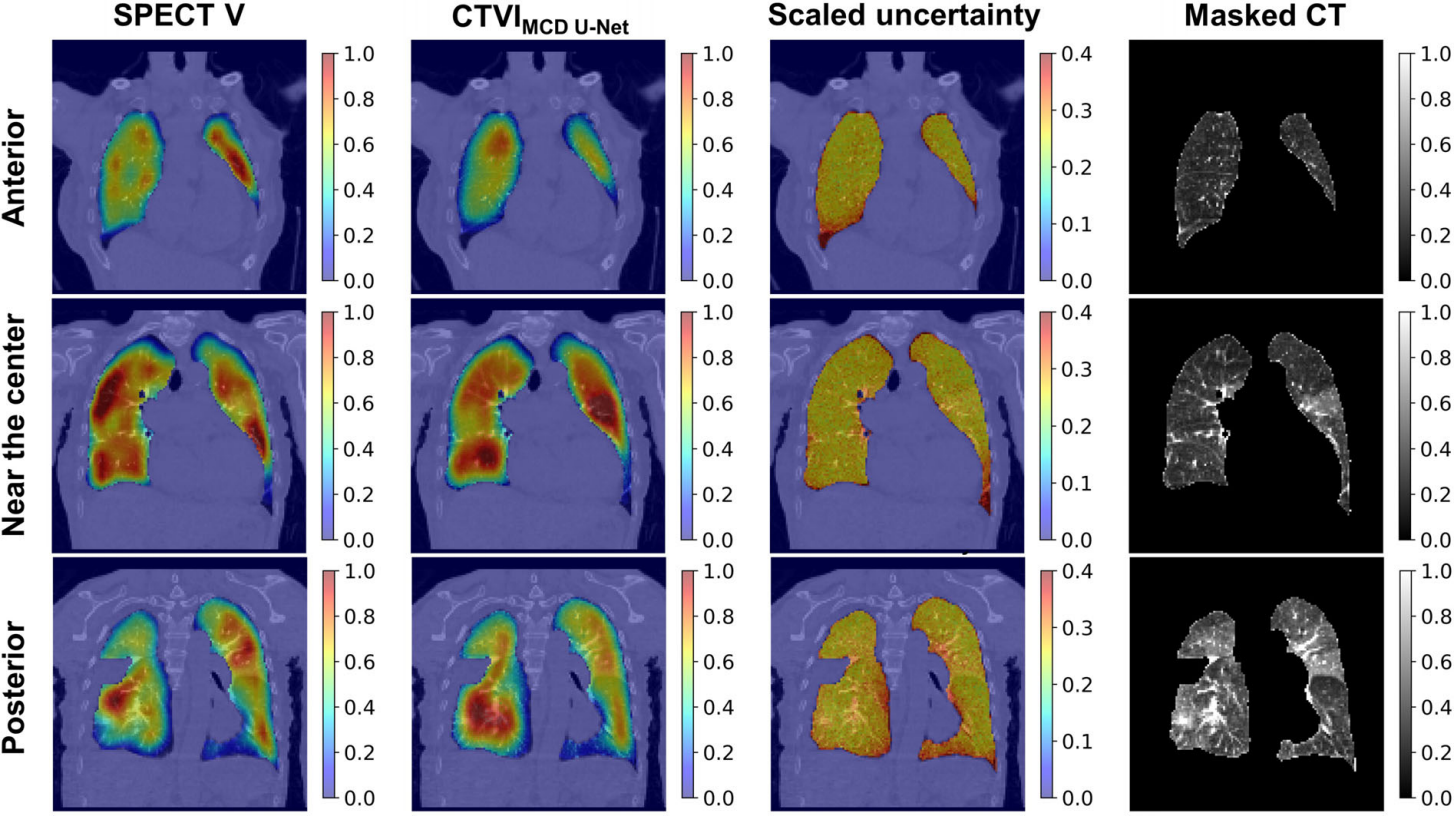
Typical cases of prediction of CTVI_MCD U‐Net_ in coronal slices. The anterior slice is shown on the top row, followed by the near, center, and posterior slices. The SPECT V is shown on the left, followed by CTVI_MCD U‐Net_, the scaled uncertainty for the CTVI_MCD U‐Net_, and masked CT. The ventilation values for viewing were normalized to give the 99th percentile value of 1 and 1st percentile value of 0. CT, computed tomography; CTVI, computed tomography ventilation imaging; MCD, Monte Carlo dropout; SPECT V, single‐photon emission computed tomography ventilation
